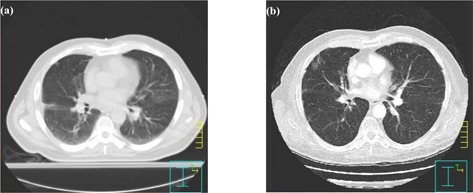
RTP and (b) contrast‐enhanced diagnostic CT scans for one of the patients in the study. These highlight some of the main differences between the scanning protocols, including the curved couch surface and an increase in the lung volume for the diagnostic scan. The presence of the contrast is also clear in the mediastinum on the diagnostic contrast‐enhanced CT scan.
